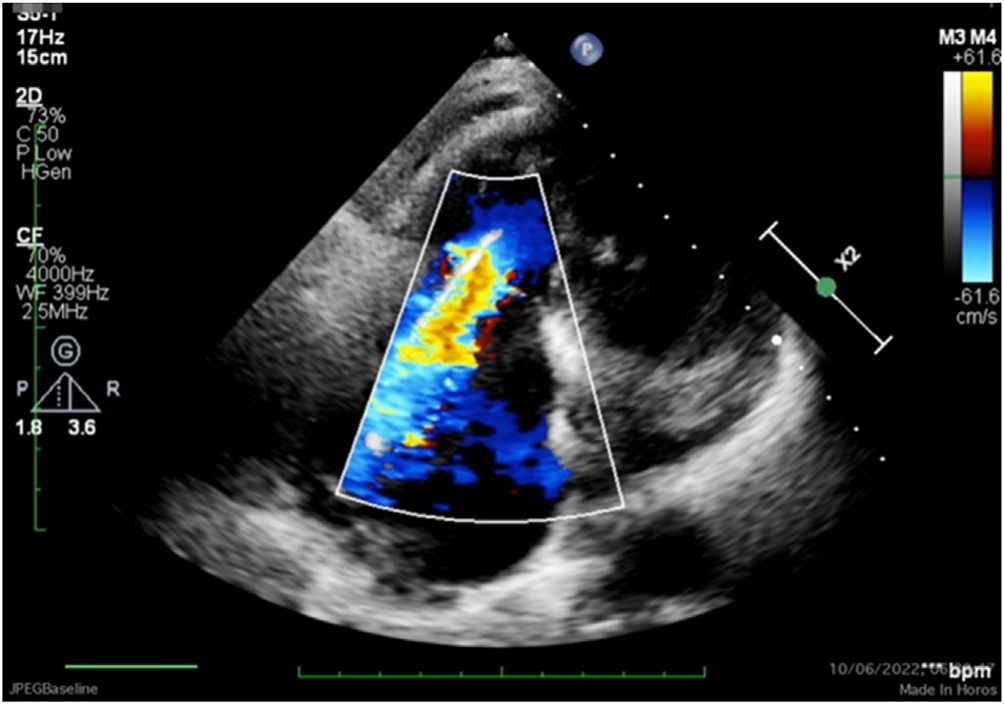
Baseline transthoracic echocardiography images demonstrating the broad vena contracta (apical RV-focused 4 chamber) view. Abbreviation: 2D, 2-dimensional; bpm, beats per minute; CF, color flow; RV, right ventricle.
